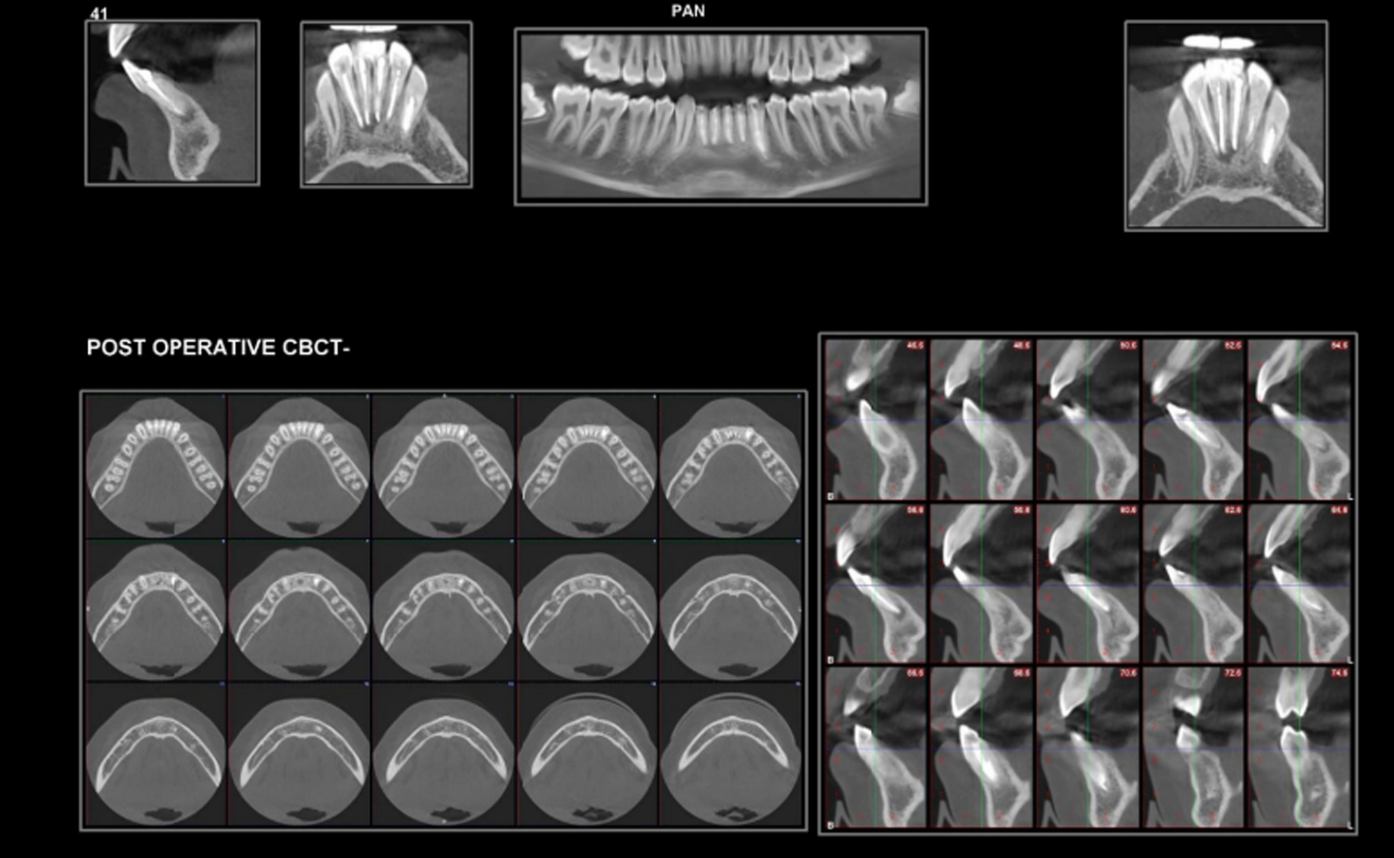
Two‐year follow‐up—CBCT.
